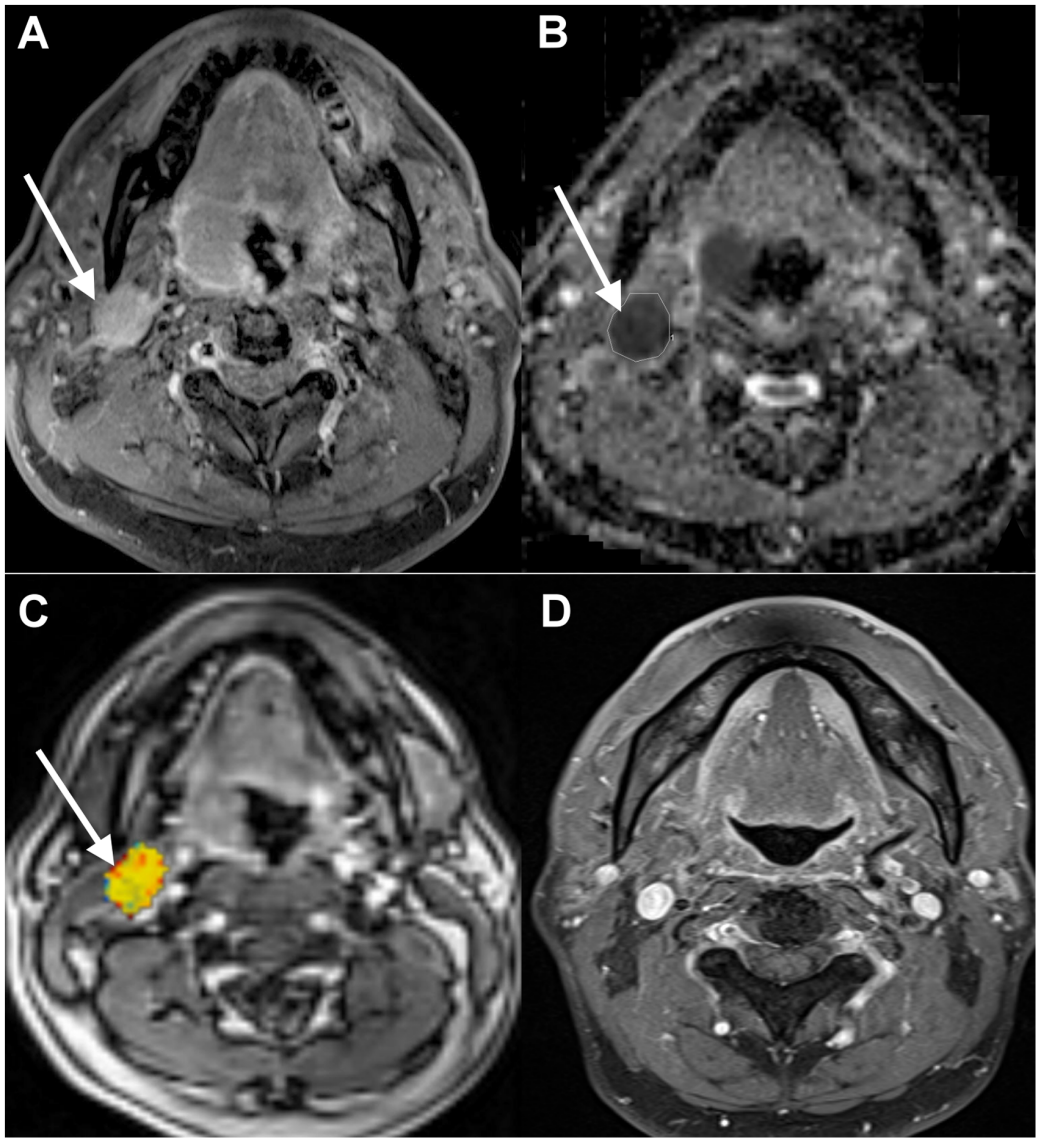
Neck control in a 53-year-old male patient with right tonsillar oropharyngeal SCC after chemoradiation. The patient’s hemoglobin level was 15.9 g/dL. A. Pretreatment axial-enhanced MRI identified a right cervical metastatic node (arrow). B. The corresponding ADC map showed a nodal ADC value of 0.78×10^−3^ mm^2^/s. C. The corresponding PWI map showed a nodal *V*
